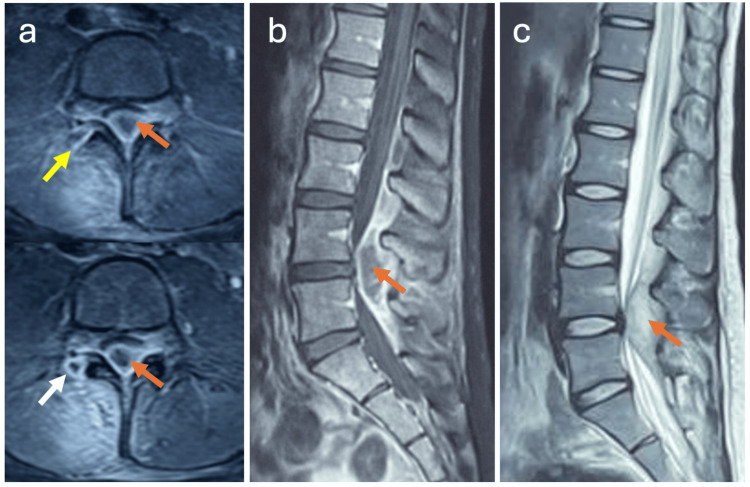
MRI of the lumbosacral spine showing a typical rim-enhancing epidural collection contiguous with the right L4-L5 SAFJ (Case 2). Axial fat-suppressed contrast-enhanced T1-weighted images (a) demonstrate enhancement of the right L4-L5 facet joint (yellow arrow) with a rim-enhancing lesion (white arrow) involving the articular process and an associated compressive epidural abscess. Sagittal contrast-enhanced T1-weighted image (b) and sagittal T2-weighted image (c) show a posterior epidural abscess (orange arrow) extending from L4 to L5, resulting in significant compression of the thecal sac and cauda equina nerve roots. MRI, magnetic resonance imaging
